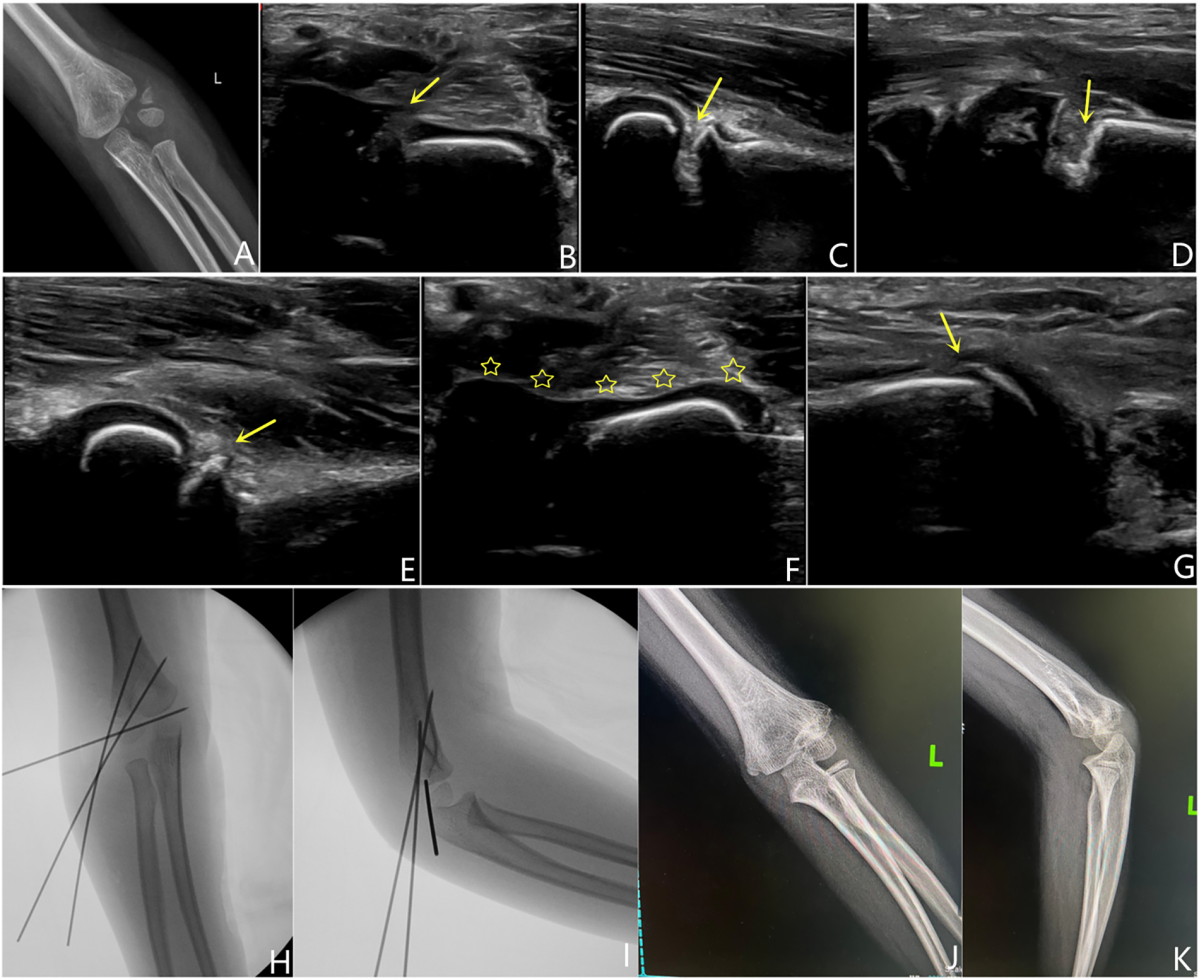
Boy, 5 years old, with left humeral lateral condyle fracture, song type 5. (A) Preoperative x-ray showed a rotated fragment (arrow). (B–D) Before reduction, severe displacement of the fracture can be seen on ultrasound in the coronal, sagittal, and metaphyseal directions (arrow). (E–G) After reduction and fixation, fracture reduction can be seen in the sagittal, coronal, and metaphyseal directions on ultrasound, with continuous cartilage hinge. (H,I) Postoperative imaging showed continuous cartilage hinge and divergent Kirschner wires fixation after reduction. (J,K) After 21 months of surgery, the follow-up pictures showed that the fracture were normal-like shape with minor lateral spur.
